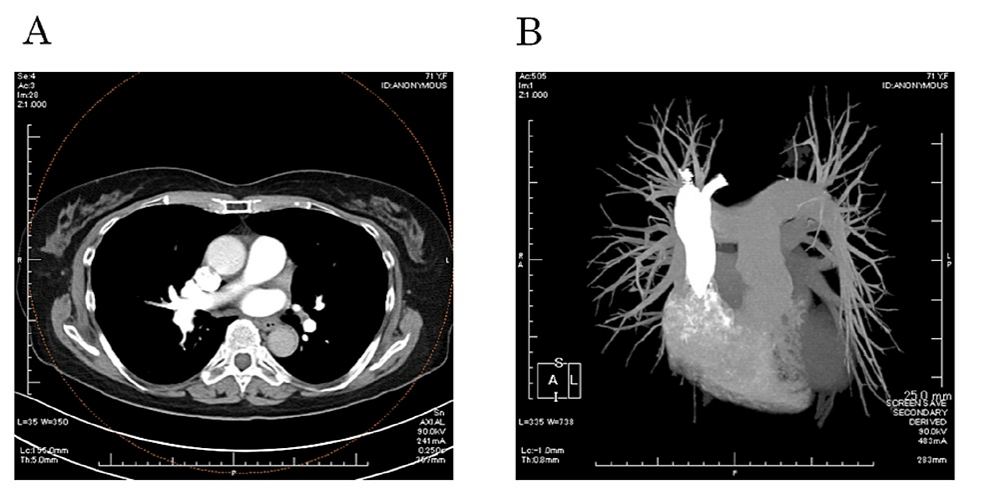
Computed tomography angiography of the pulmonary arteries on day 9. The thrombi were resolved. A. An axial image. Thrombi were resolved compared with that at baseline. B. Three-dimensional computed tomography angiography did now show a thrombus in the pulmonary arteries.
